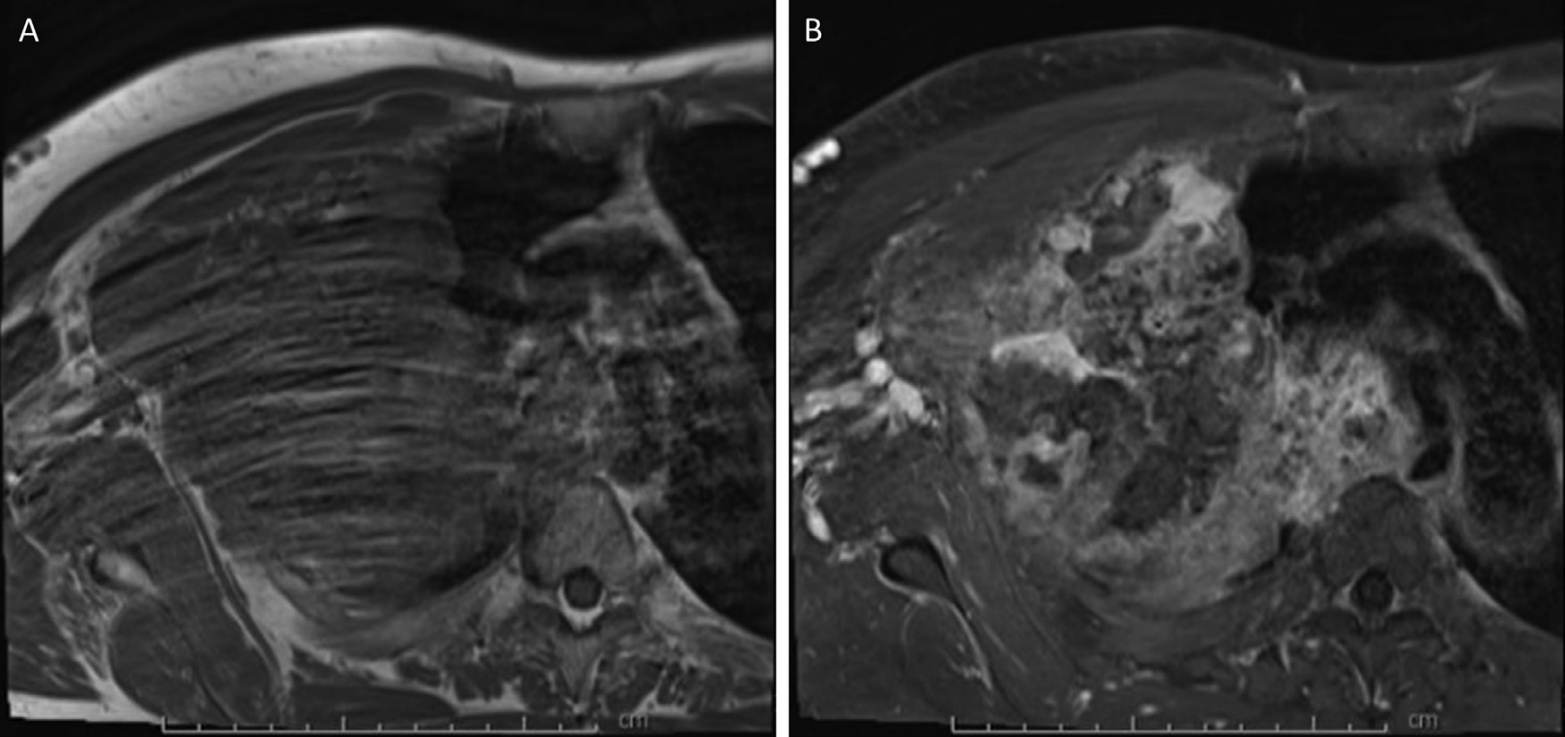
MRI brachial plexus protocol, T1 axial (A), and T1 post gadolinium (B) demonstrates a T1 isointense peripherally enhancing right upper chest wall mass extending into the right axilla, and eroding right second rib
